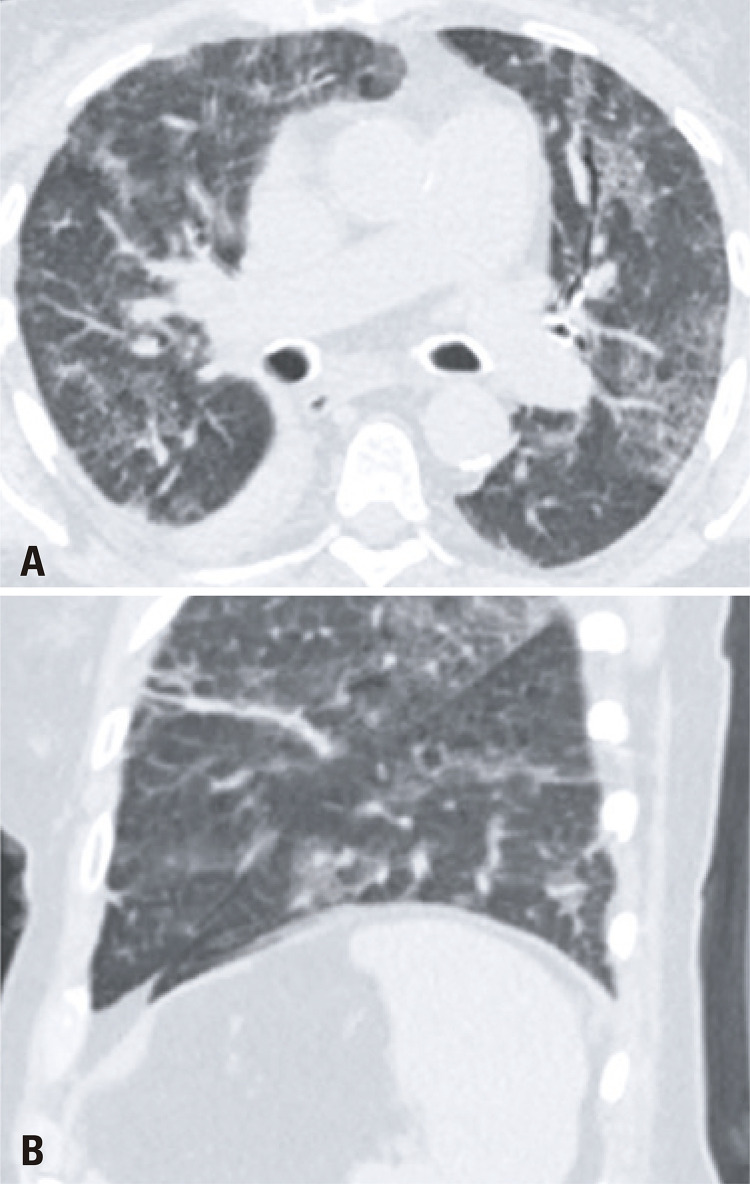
Axial (A) and sagital (B) images of the lung basis in an abdomen computed tomography show ground-glass opacities with septal thickening and fine reticulation, typical COVID-19 pulmonary findings
